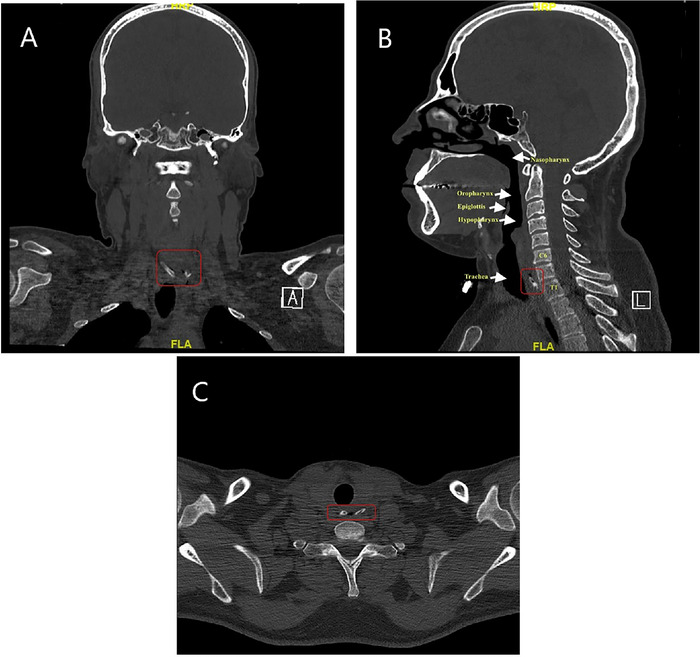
(A) Coronal, (B) sagittal, and (C) cross-sectional computed tomography scans of the head and neck show the impacted foreign body [red boxes].
